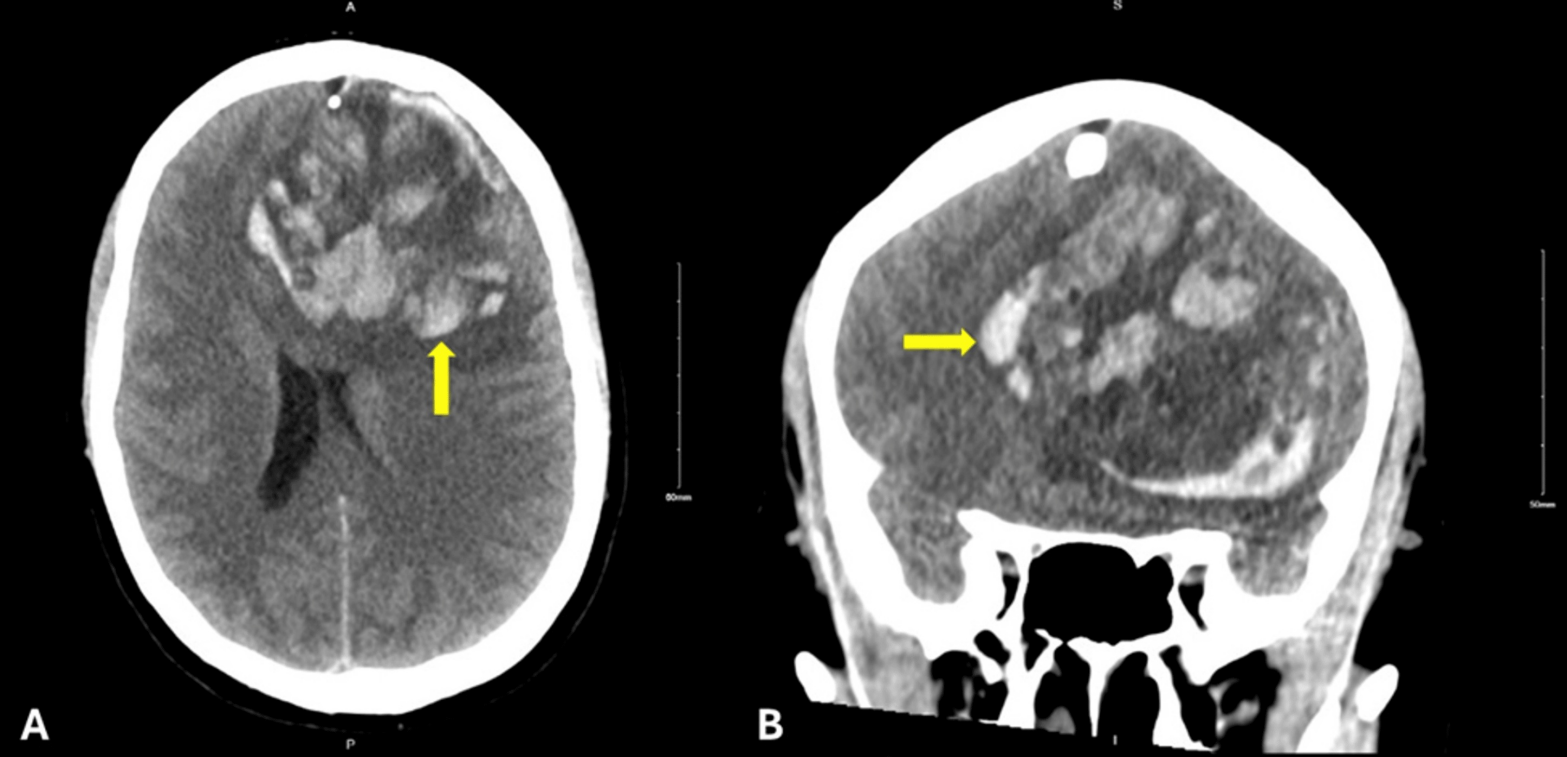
Brain metastasis with hemorrhage. Cross-sectional computed tomography imaging of the head without IV contrast. (A) Axial and (B) coronal views displaying a hemorrhagic lesion 7x7x5.5 cm (yellow arrow) in the left frontal lobe with subfalcine herniation, left uncal herniation, and midline shift.
